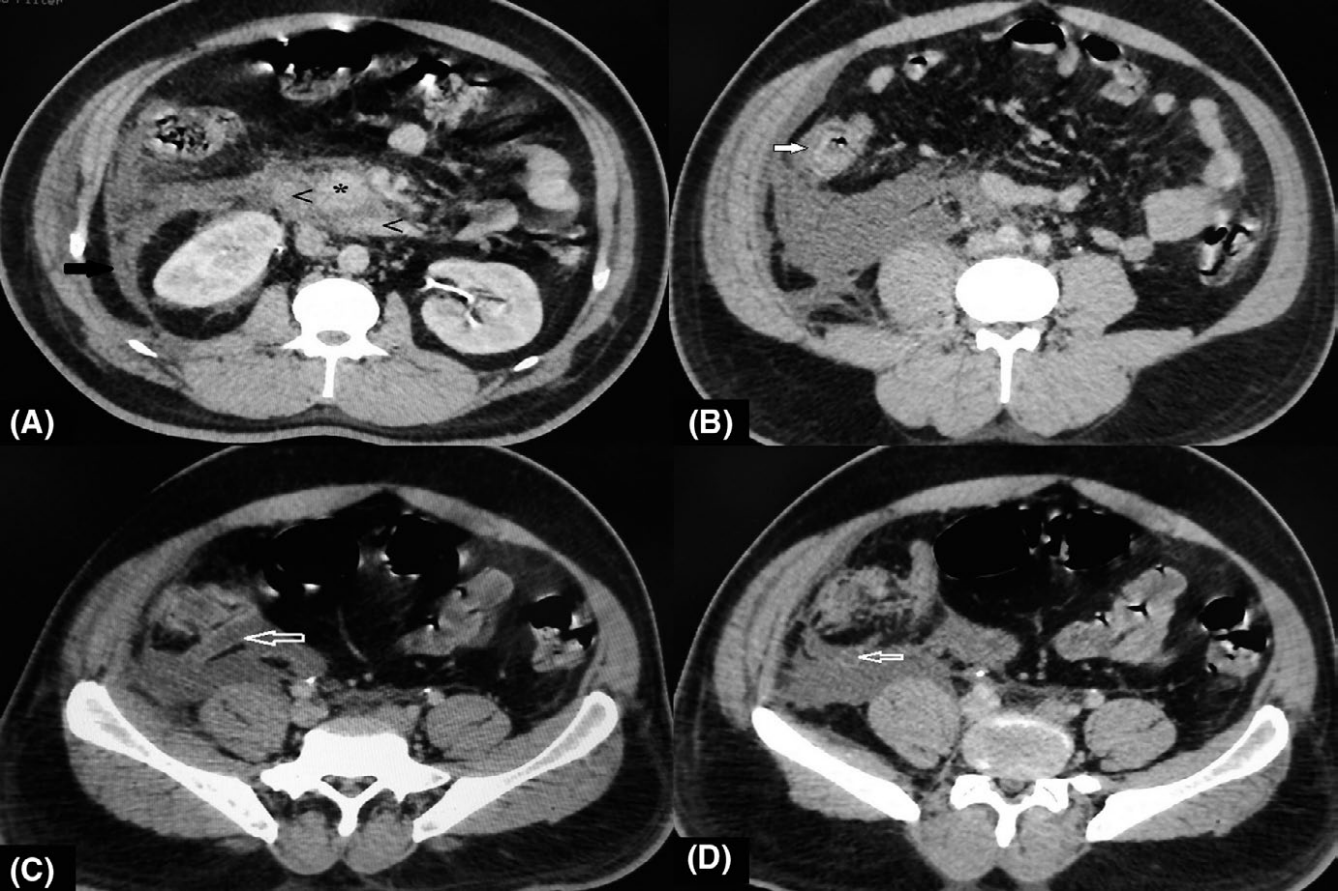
Acute pancreatitis and acute appendicitis. Contrast CT of abdomen: (A) demonstrates strandings along the uncinate process of pancreas (*) along with fluid collection in peripancreatic and periduodoneal (>> second and third part of duodeneum) region of retroperitoneum. Fluid in pararenal retroperitoneum in right side limited posteriorly by lateralconal fascia (black arrow). (B) Mural stratification of caecum with symmetrical regular circumferental edematous wall. Fluid collection in retroperitoneum is displacing caecum anteriorly. (C, D) demonstrates retrocaecal appendix is distended (11 mm) with fluid and with mild enhancing smooth wall and with periappendiceal fluid collection
